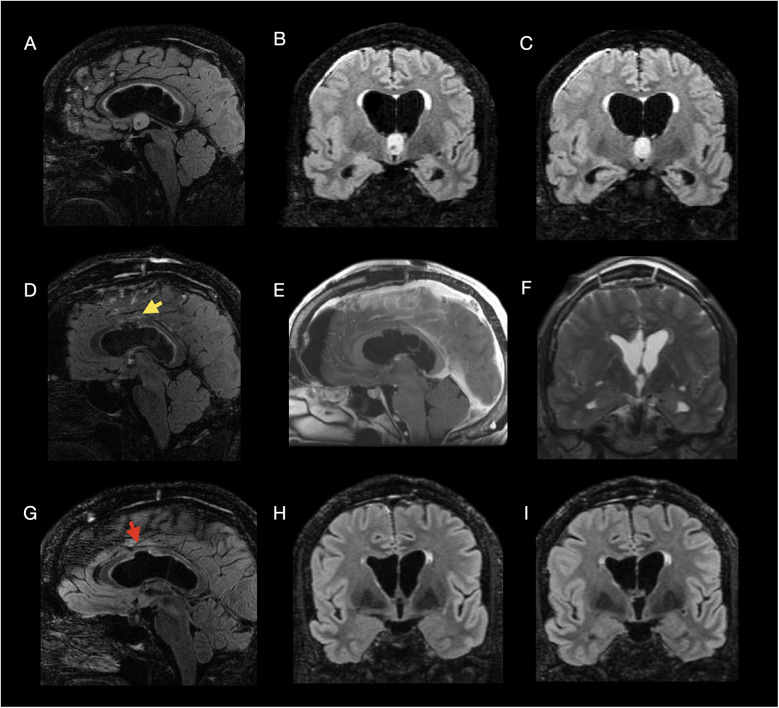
Multimodal imaging of a 64-year-old male with headache due to a third ventricular colloid cyst, treated via open interhemispheric transcallosal resection. Preoperative images (A–C): Sagittal (A) and coronal (B,C) 3D T2-weighted FLAIR images demonstrate a heterogeneously enhancing lesion measuring approximately 1.6 cm in maximum diameter at the superior margin of the foramen of Monro, consistent with a colloid cyst, causing moderate dilation of the lateral ventricles. Postoperative day 1 MRI (D–F): Sagittal 3D T2-weighted FLAIR (D), sagittal post-contrast T1-weighted (E), and coronal 3D T2-weighted FLAIR (F) images show expected postoperative changes without residual lesion, and minimal hyperintensity along the corpus callosotomy tract. FouR,Month folloW,Up MRI (G–I): Sagittal (G) and coronal (H,I) 3D T2-weighted FLAIR images show no recurrence and demonstrate the callosotomy defect without additional parenchymal tissue loss. Arrow key: Yellow arrow: postoperative hyperintensity (D); red arrow: callosotomy defect (G).
